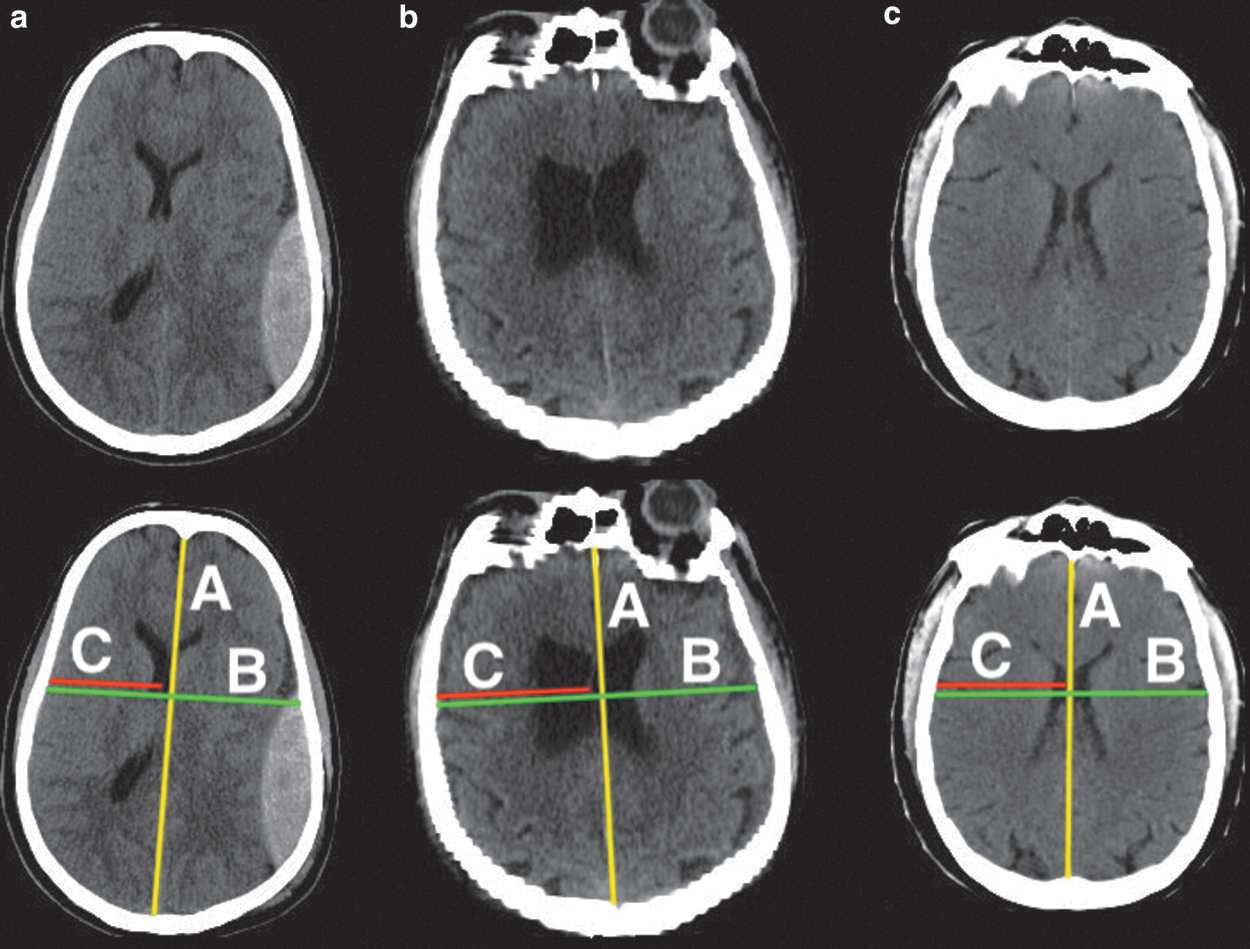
Qualitative results for midline shift estimation on 3 representative subjects from data set 3. First row presents original computed tomography images and second row shows how the midline is calculated by the expert. The estimated shifts (in mm) by the expert and icobrain for (A) is (5.60, 5.95), followed by (1.10, 1.91) for (B) and (0.40, 1.91) for (C). Color image is available online.
